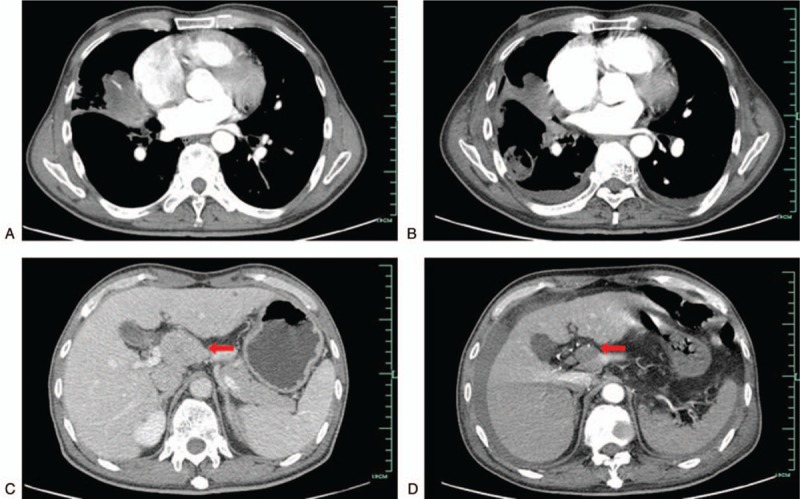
Computed tomograhy presented the changes of lesions before and after Apatinib treatment. A/C: primary pulmonary inflammatory myofibroblastic tumor (IMT) (A) and intra-abdominal metastasis (C) before Apatinib treatment. B/D: primary pulmonary IMT (B) and intra-abdominal metastasis (D) after Apatinib treatment for 5 months.
